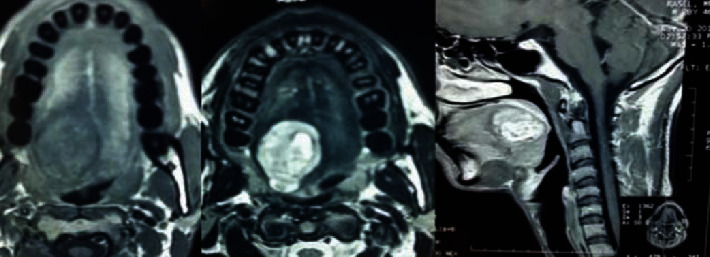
Axial T1 and T2 and sagittal T1-weighted magnetic resonance image showing a well-defined mass centered right to the midline in the base of the tongue. The tumor has a smooth well-defined border, with no invasion.
